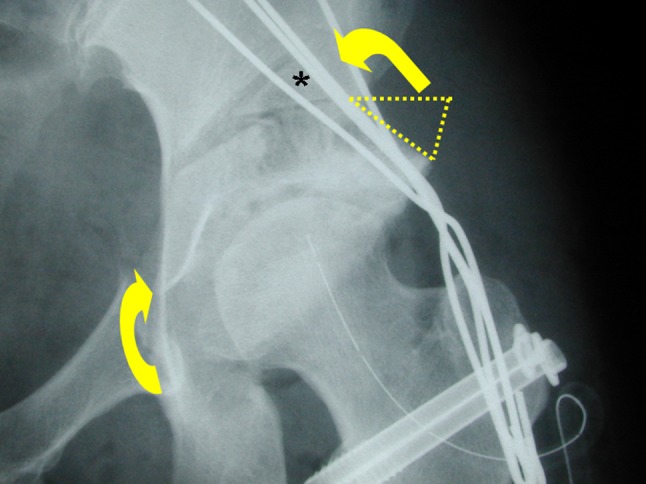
An AP postoperative radiograph of the left hip shows the osteotomized acetabulum is rotated anterolaterally (lower arrow) with a trapezoid-shaped bone graft (asterisk) from the lateral part of the osteotomized fragment (upper arrow).
